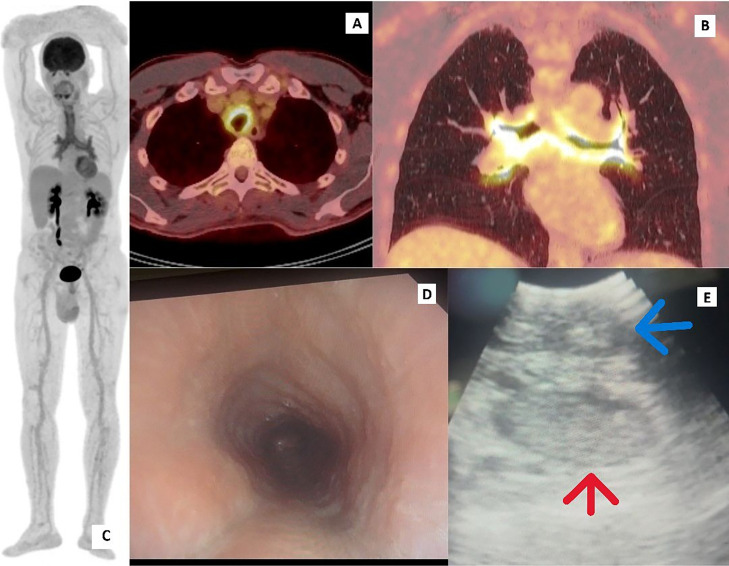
A, PET-CT scan demonstrating uptake of 18-FDG by the tracheal wall, sparing the posterior tracheal circumference (transverse view). B, 18-FDG uptake of the main and proximal bronchi symmetrically (coronal view). C, A full-body PET-CT scan showing the diffuse uptake of 18-FDG by the trachea and main bronchi. D, Bronchoscopic image of the trachea demonstrating diffuse mucosal oedema, sparing the posterior tracheal circumference. E, An EBUS image showcasing the thickened tracheal cartilage (blue arrow) and an enlarged paratracheal lymph node (red arrow). EBUS: Endobronchial Ultrasound 18-FDG: fluorine-18 fluorodeoxyglucose.
